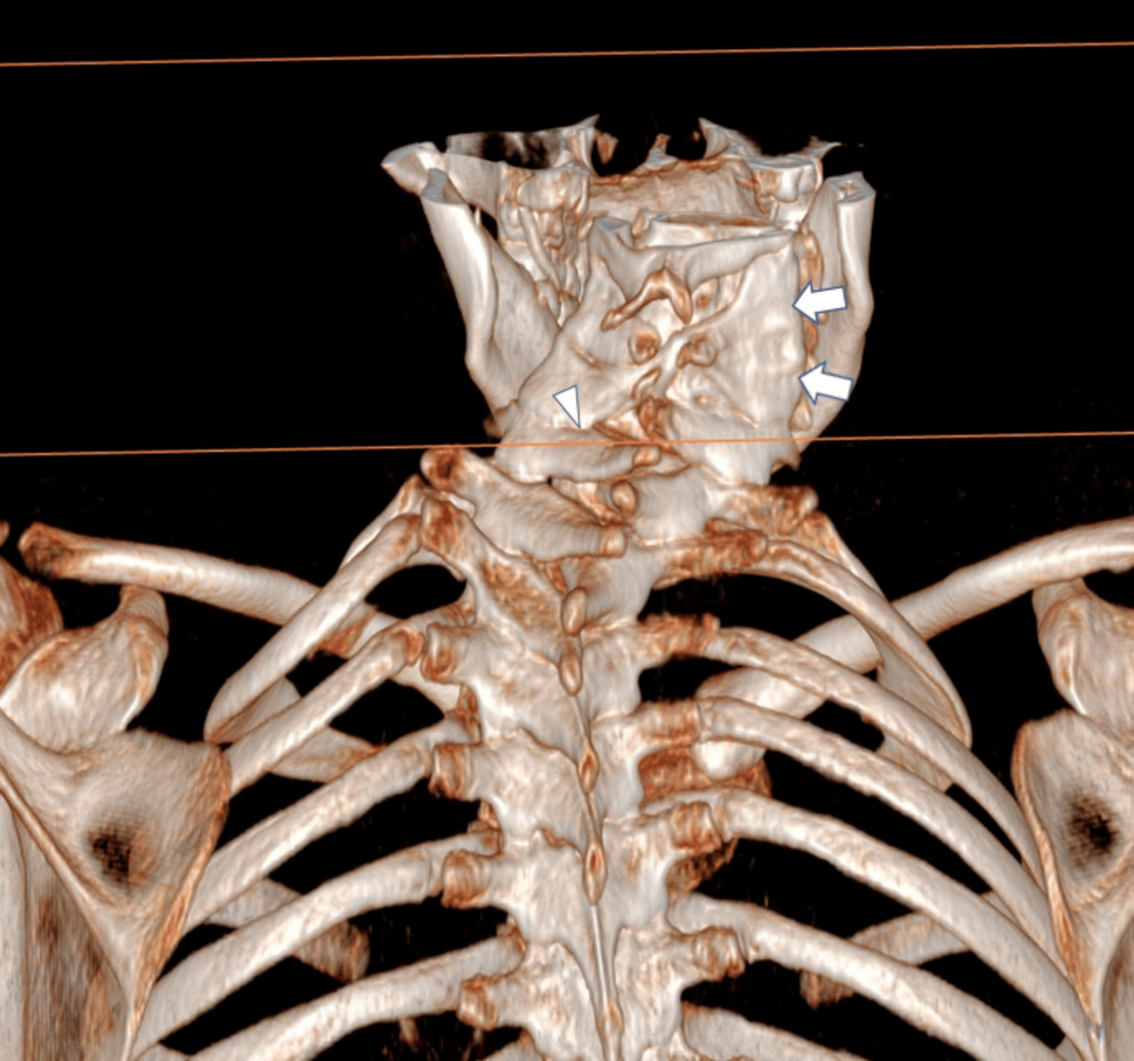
Volume rendering three-dimensional CT lateral cervical image showing the fusion of the C3-C4-C5 vertebral body (arrow) with partial posterior fusion of the C5-C6 vertebral body (arrowhead).
